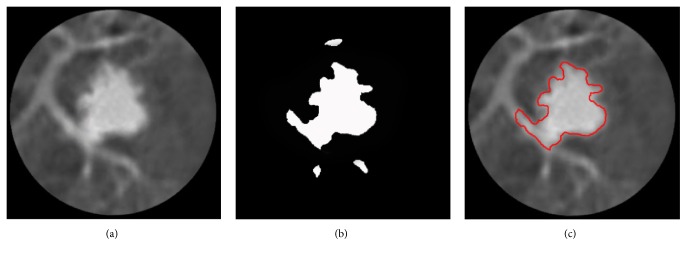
The result of initial contour. (a) ROI of pulmonary nodule; (b) binary CT image; (c) initial contour.
